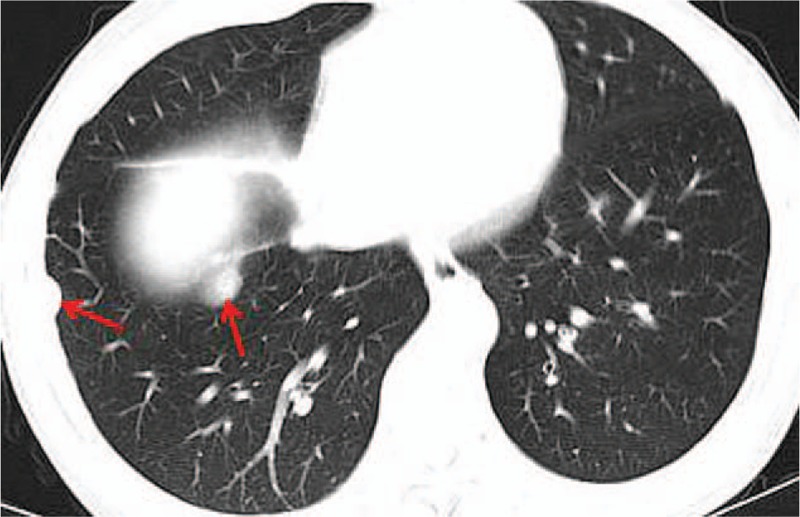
The contrast-enhanced CT revealed recurrence in the patient's chest wall and right middle lung (arrows).
